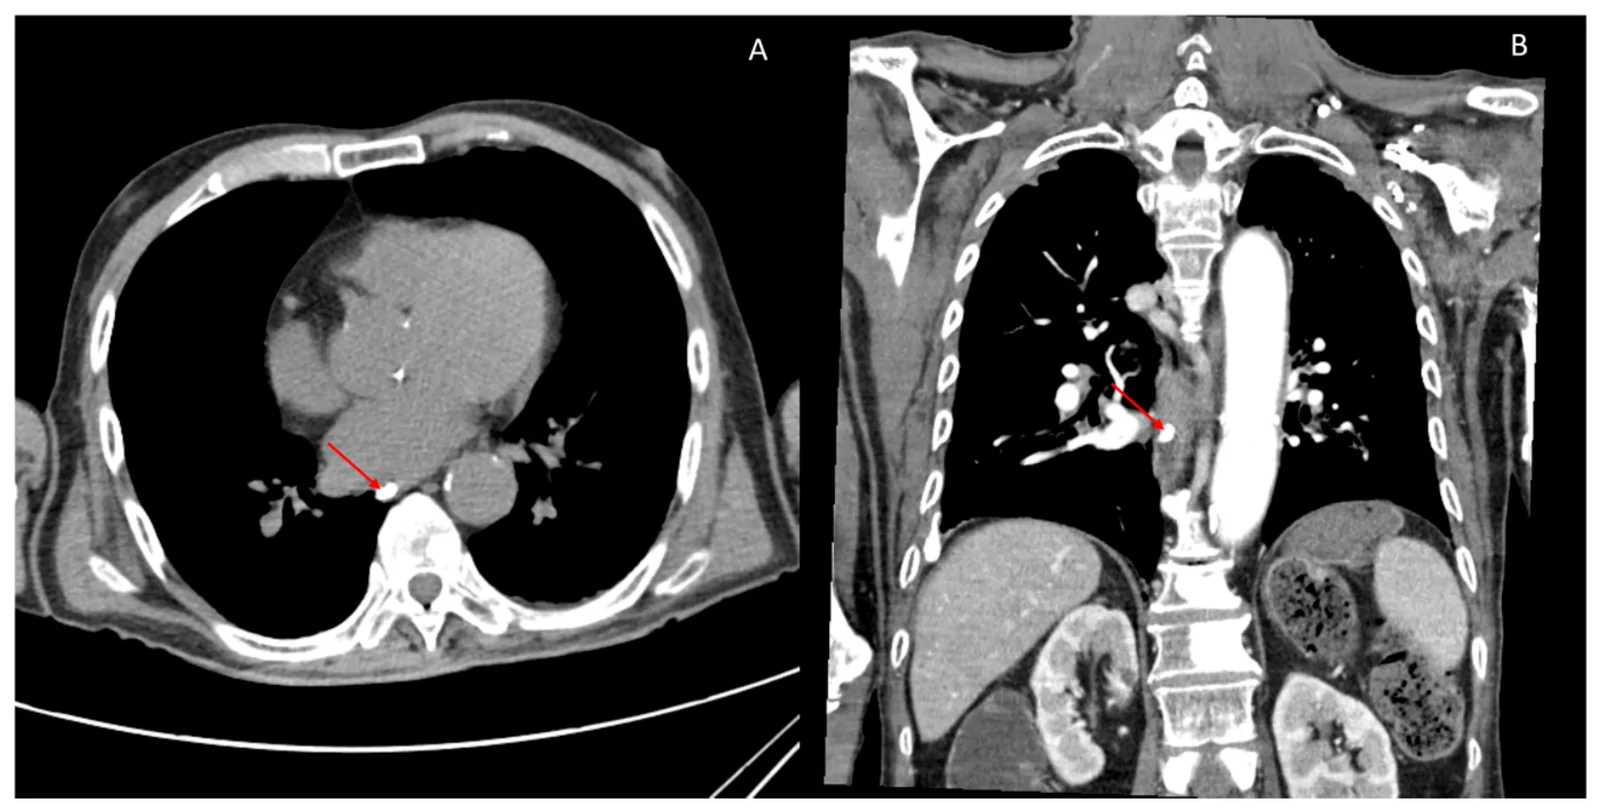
Chest computed tomography demonstrating a tooth-like radiopaque foreign body in the lower esophagus. (A) Axial CT image. (B) Coronal reconstructed CT image. The red arrows indicate the suspected tooth-like foreign body.
